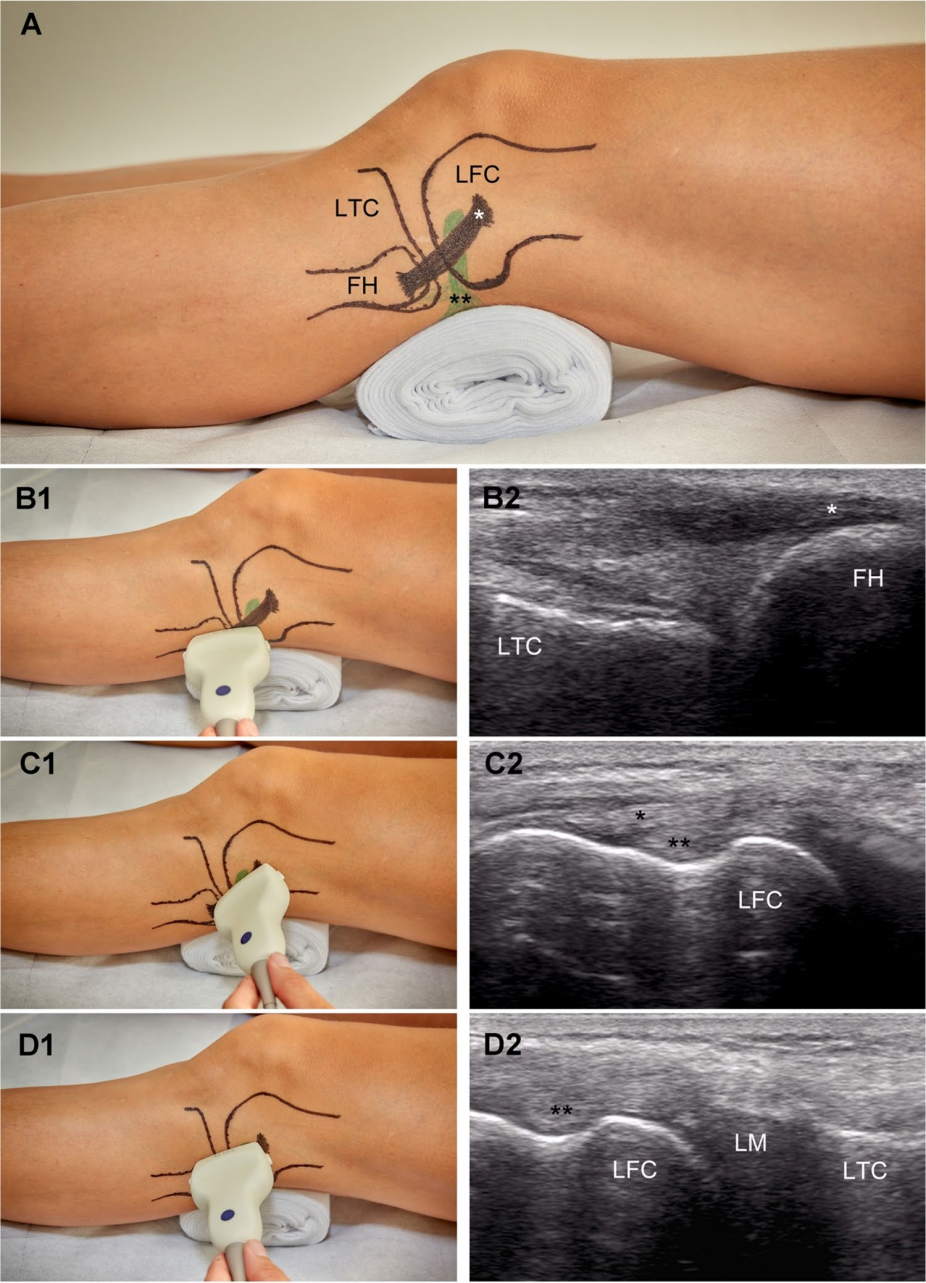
Standardized ultrasound-based examination of lateral meniscal extrusion. a Lateral view of the knee joint depicting the relevant landmarks. b1, b2, c1, c2, d1, d2 Transducer positioning and related ultrasound image for slice 1, 2 and 3, respectively. FH, fibular head. LFC, lateral femoral condyle. *LM* lateral meniscus. *LTC* lateral tibial condyle. *, lateral collateral ligament. , popliteus tendon
